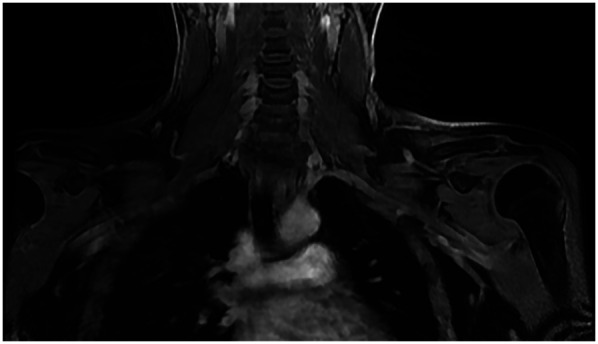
MRI of cervical spine (sagittal T2-weighted turbo spin-echo). The image shows reversed cervical lordosis (straightened curvature). Critically, no spinal cord compression, disc herniation, or nerve root avulsion is observed, which helped exclude structural cervical spine pathology as the cause of the patient's neurological deficits.
